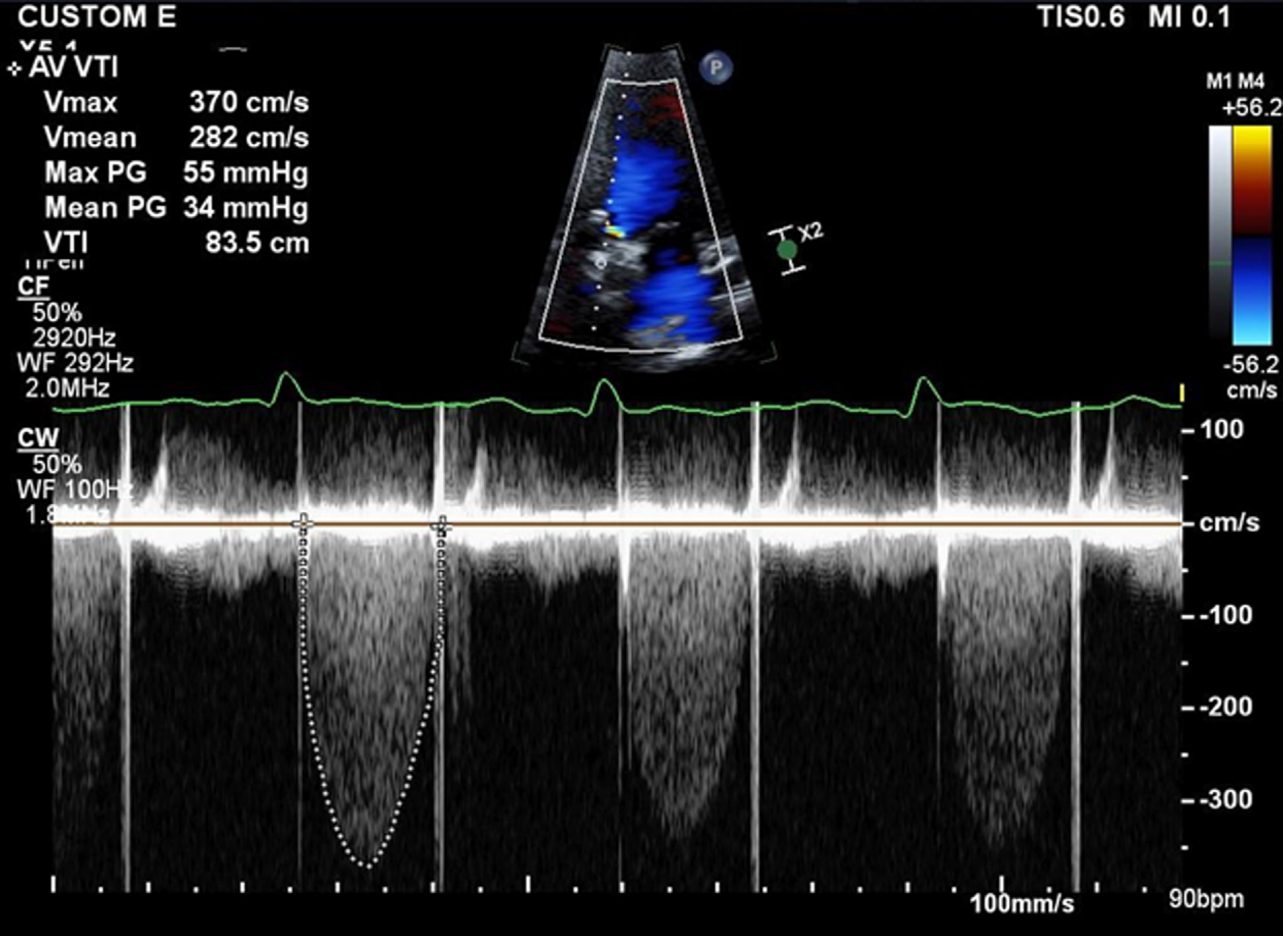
Transthoracic Echocardiography Before Tissue Plasminogen Activator Transthoracic echocardiography showing elevated peak and mean aortic transvalvular gradient of 55 mm Hg and 34 mm Hg, respectively.
